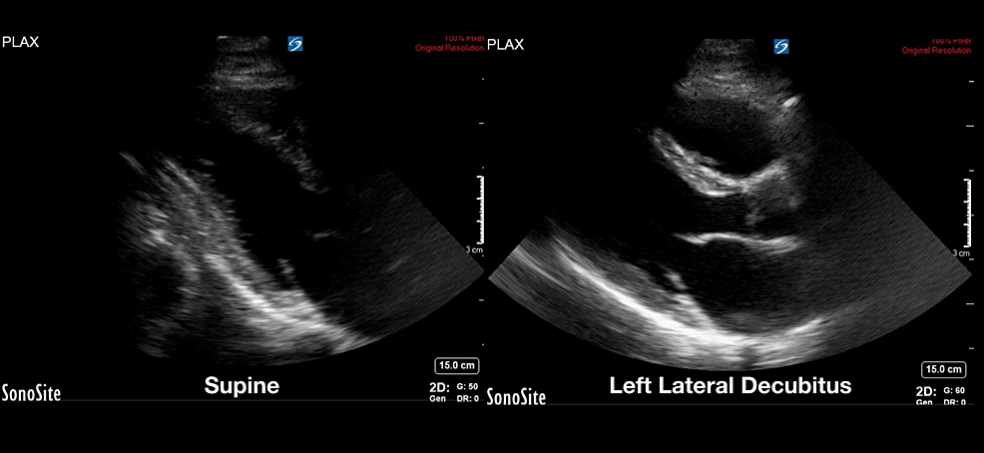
Example of image quality difference in the parasternal long window between the supine and left lateral decubitus positions within the same patient
